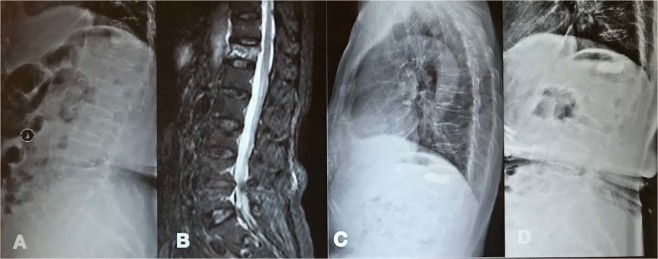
A 61-year-old female patient with an L1 osteoporotic fracture. T12 was treated surgically with PV. (AB) Preoperative X-ray and MRI demonstrated a T12 fracture, and the preoperative ASA and TLA were 29.64° and 25.60° on plain radiography and 8.31° and 15.66° on MRI, respectively. She underwent PV. (CD) At the 3-month FU and 4-year FU, the X-ray showed that the ASA and TLA worsened. L1 fracture was observed.
